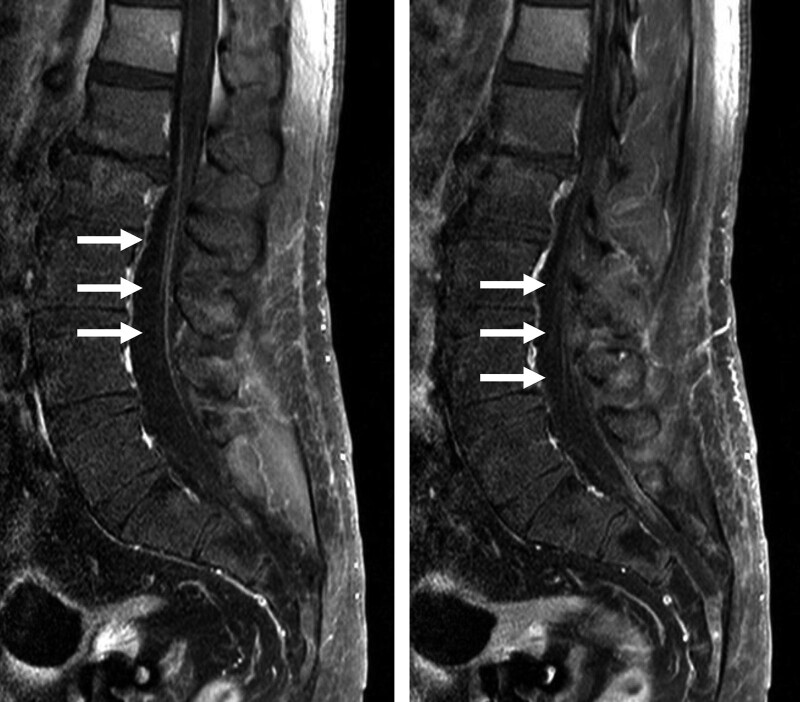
Enhancement of cauda equinae is observed on T1-weighted contrast-enhanced images.
